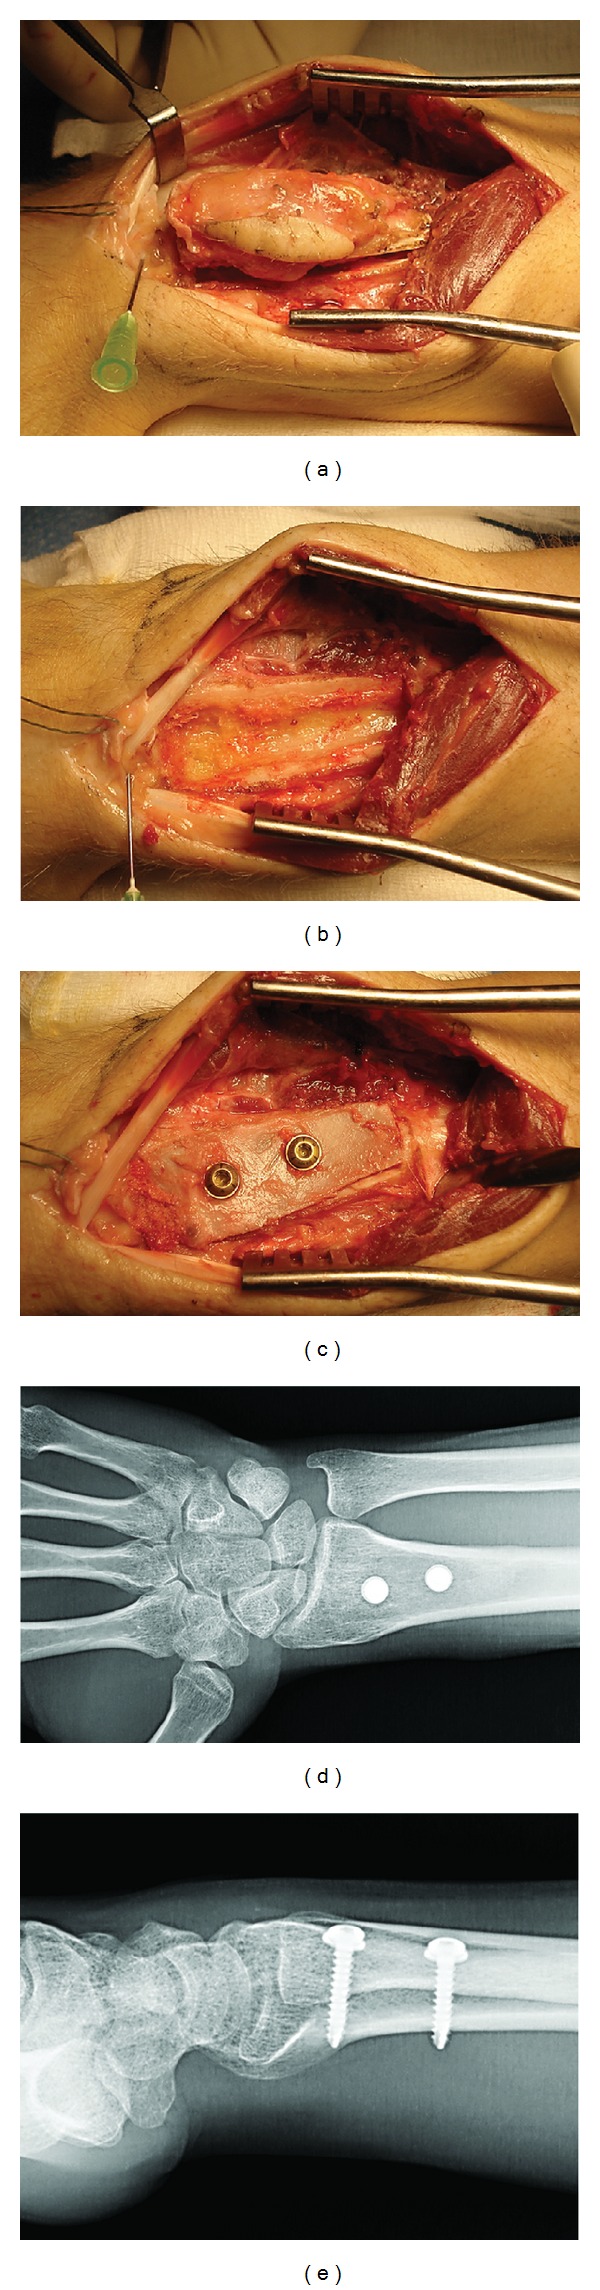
((a)–(c)) Intraoperative images. (a) En bloc resection of the biopsied area, soft tissue neoplasm, and osseous lesion. (b) Transverse semicircular osteotomy and curettage of the distal radial metaphysis. (c) Iliac crest allograft stabilized with two 3.5 mm. screws. ((d)-(e)) AP and lateral radiographs of the wrist three years after operation showing complete graft incorporation.
